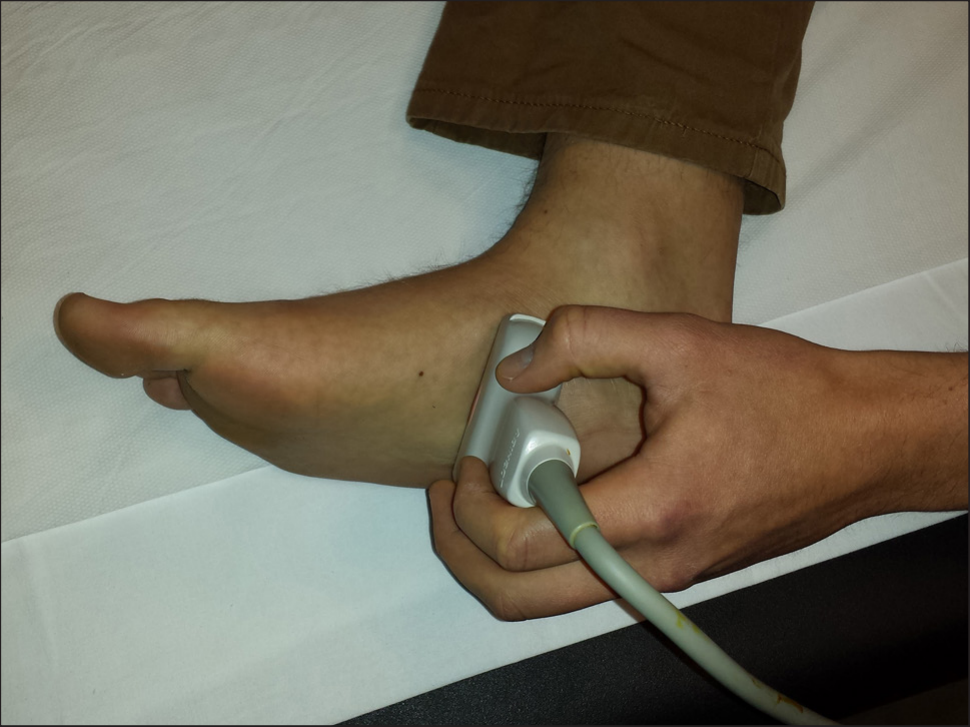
Position of the ultrasound probe for visualization of the medial plantar veins in a coronal plane. To visualize the medial plantar veins in a sagittal plane, the probe is turned 90° in a longitudinal direction on the foot.
